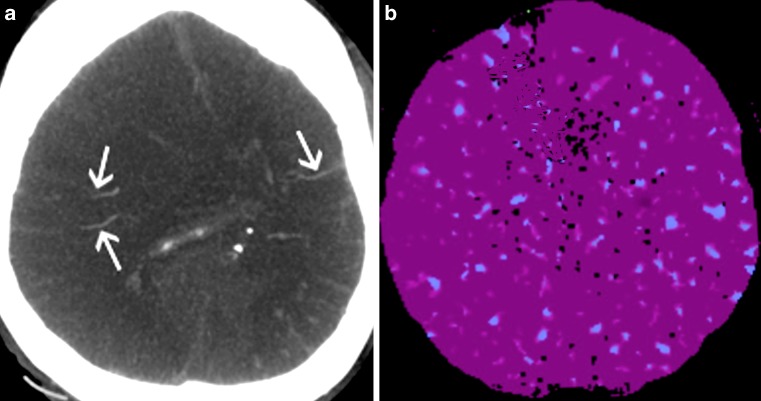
Results of CTA (a) and CTP (b) in the patient diagnosed with brain death. CTA shows filling of cortical branches of the right and left MCA (*arrows*) and was classified as negative, i. e. inconsistent with the diagnosis of BD. CTP reveals perfusion values below the thresholds for non-viable tissue and, contrary to CTA was interpreted as positive, i. e. consistent with the diagnosis of BD
